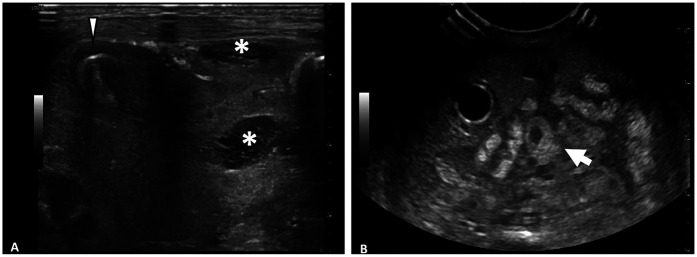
Comparison of abdominal ultrasound images obtained with high frequency vs. low frequency ultrasound transducer. (A) Image obtained with 6–15 MHz linear transducer. Improvd spatial resolution of 6–15 MHz transducer allows for visualization of fine details such as septations within fluid collections (*) and layers of intestinal wall (arrowhead). (B) Image obtained with 3–10 MHz transducer. Echogenic loop of bowel (arrow) seen, however further evaluation of intestinal wall limited by decreased spatial resolution.
